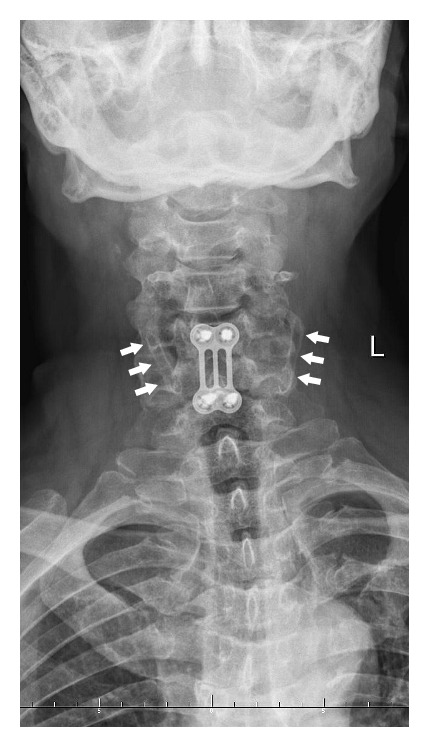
Radiograph of the cervical column showing bilateral cervical ribs (arrows) attached to the fourth cervical vertebra. Patient after neurosurgical intervention in cervical spine.
